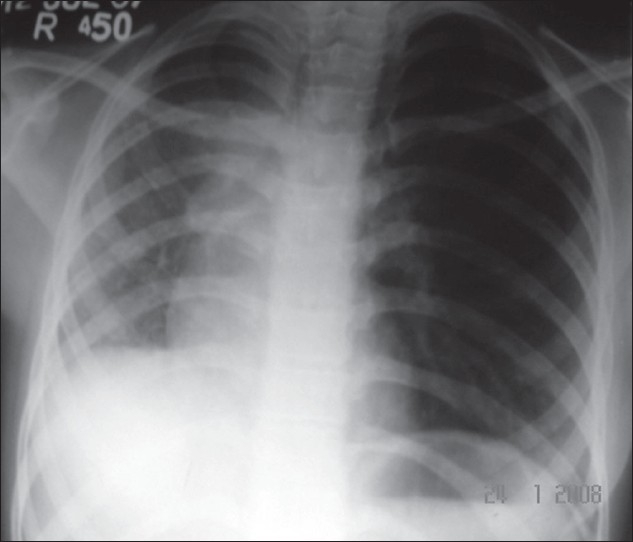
Chest radiograph showing volume loss on the right side, with shift of heart and mediastinum
